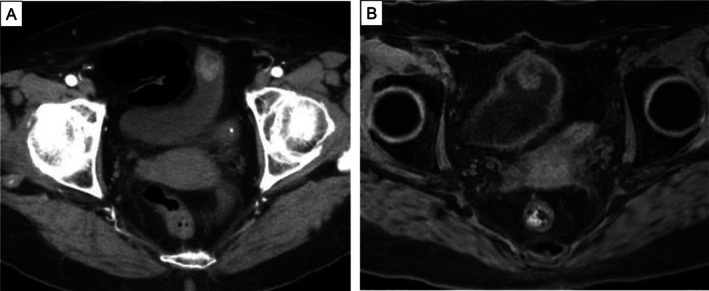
(A) Abdominal enhanced computed tomography image revealing an 18‐mm bladder tumor located on the dome of the bladder and contiguous with the umbilical ligament tumor. (B) Diffusion‐weighted magnetic resonance image showing an 18‐mm solid tumor on the dome of the bladder and exhibiting the inchworm sign.
